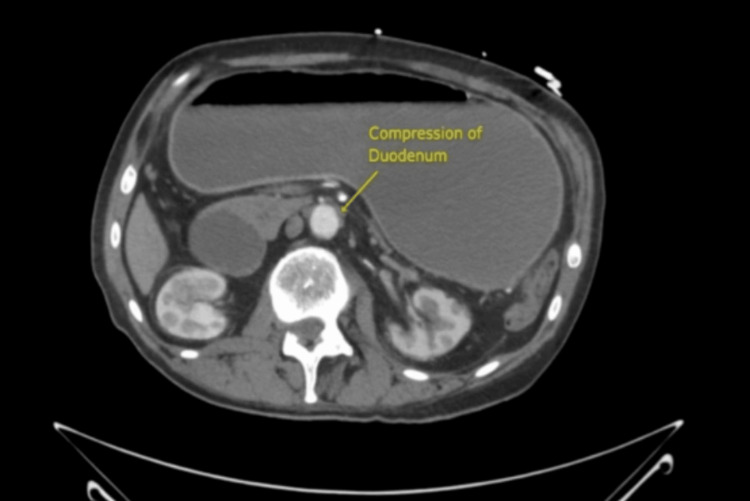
Axial contrast-enhanced CT image of the abdomen. Contrast-enhanced axial CT image demonstrating compression of the third portion of the duodenum between the SMA and the abdominal aorta (shown in yellow).
